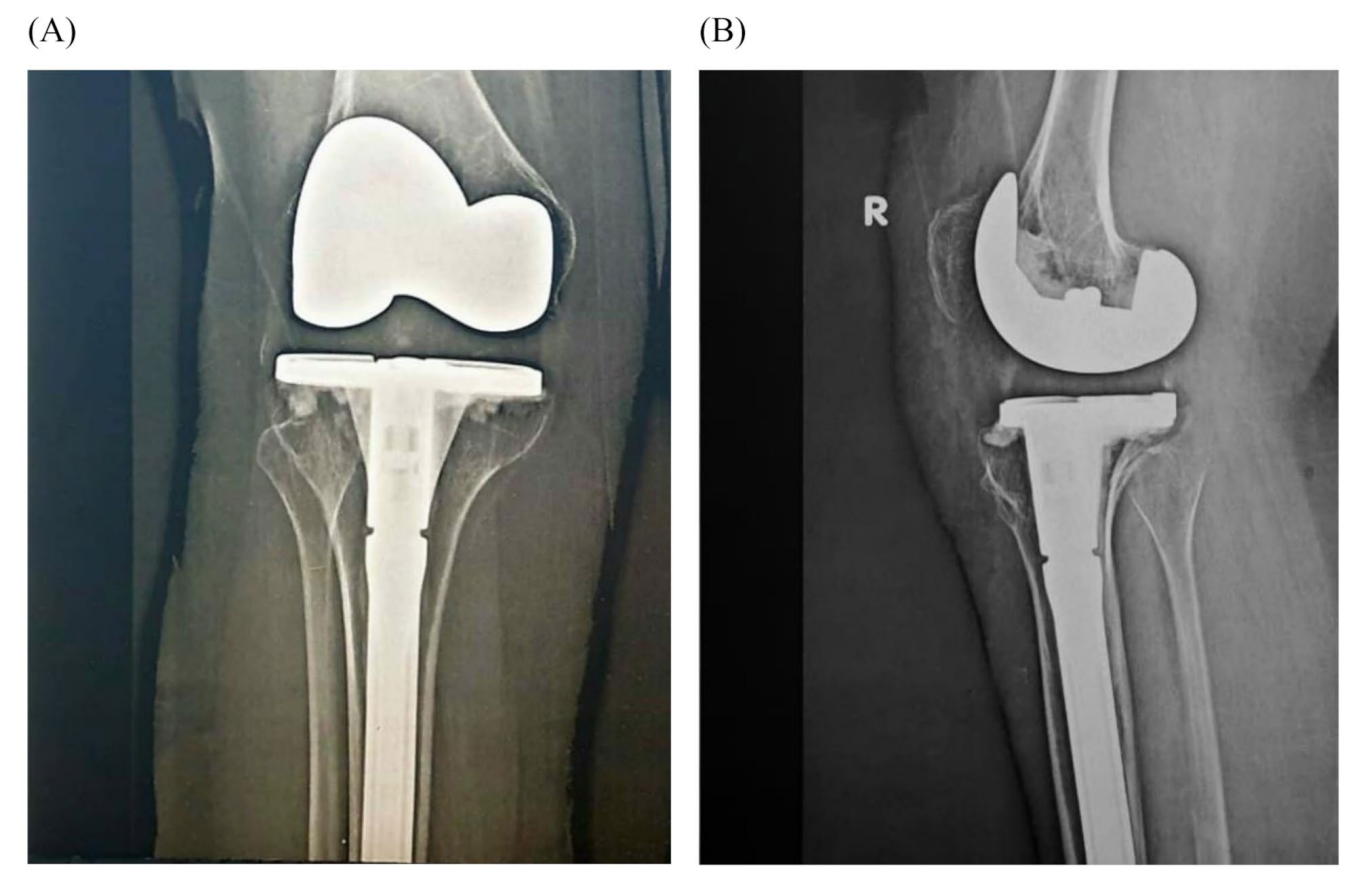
Postoperative antero-posterior (A) and lateral (B) x-rays of the right knee
